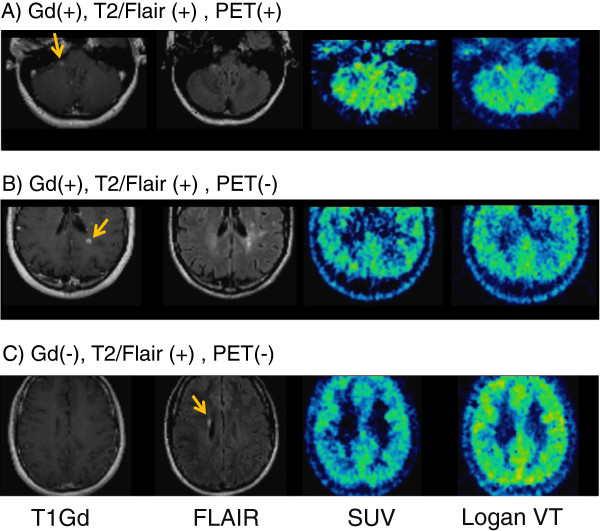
Typical MRI images and corresponding PET images for MRI-defined MS lesions. There were three patterns as follows: (A) Gd-enhanced and high intensity in T2/FLAIR images and high uptake in PET images. The images are from subject A. The arrow indicates the MS lesion. (B) Gd-enhanced and high intensity in T2/FLAIR but not high uptake in PET images. The images are from subject D. (C) Not Gd-enhanced but high intensity in T2/FLAIR and not high uptake in PET images.
